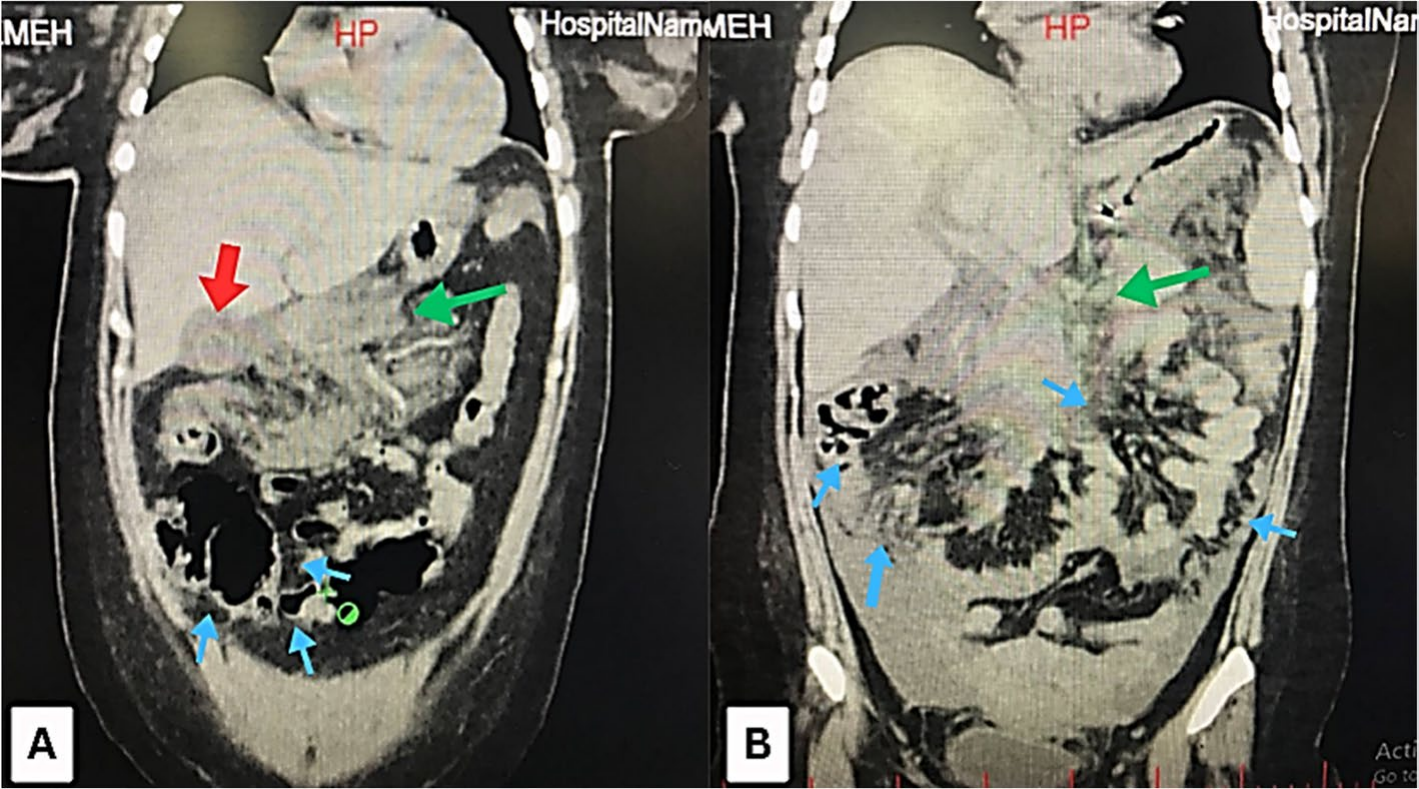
A An axial CT scan of the abdomen revealed gallstones (red arrow), evidence of pancreatitis (green arrow), and bowel dilation (blue arrows). B An axial CT scan of the abdomen showed extensive pancreatic inflammation (green arrows), additionally, (blue arrows) indicate bowel edema and peritoneal fluid accumulation, suggestive of peritonitis."
